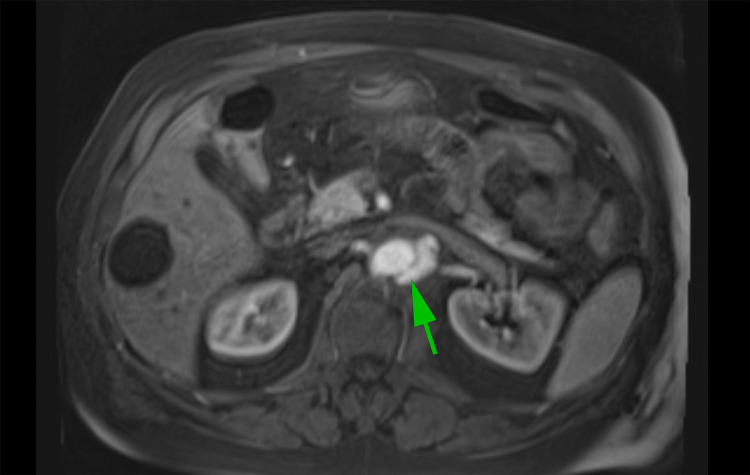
Magnetic resonance imaging revealed a mycotic abdominal aortic aneurysm
